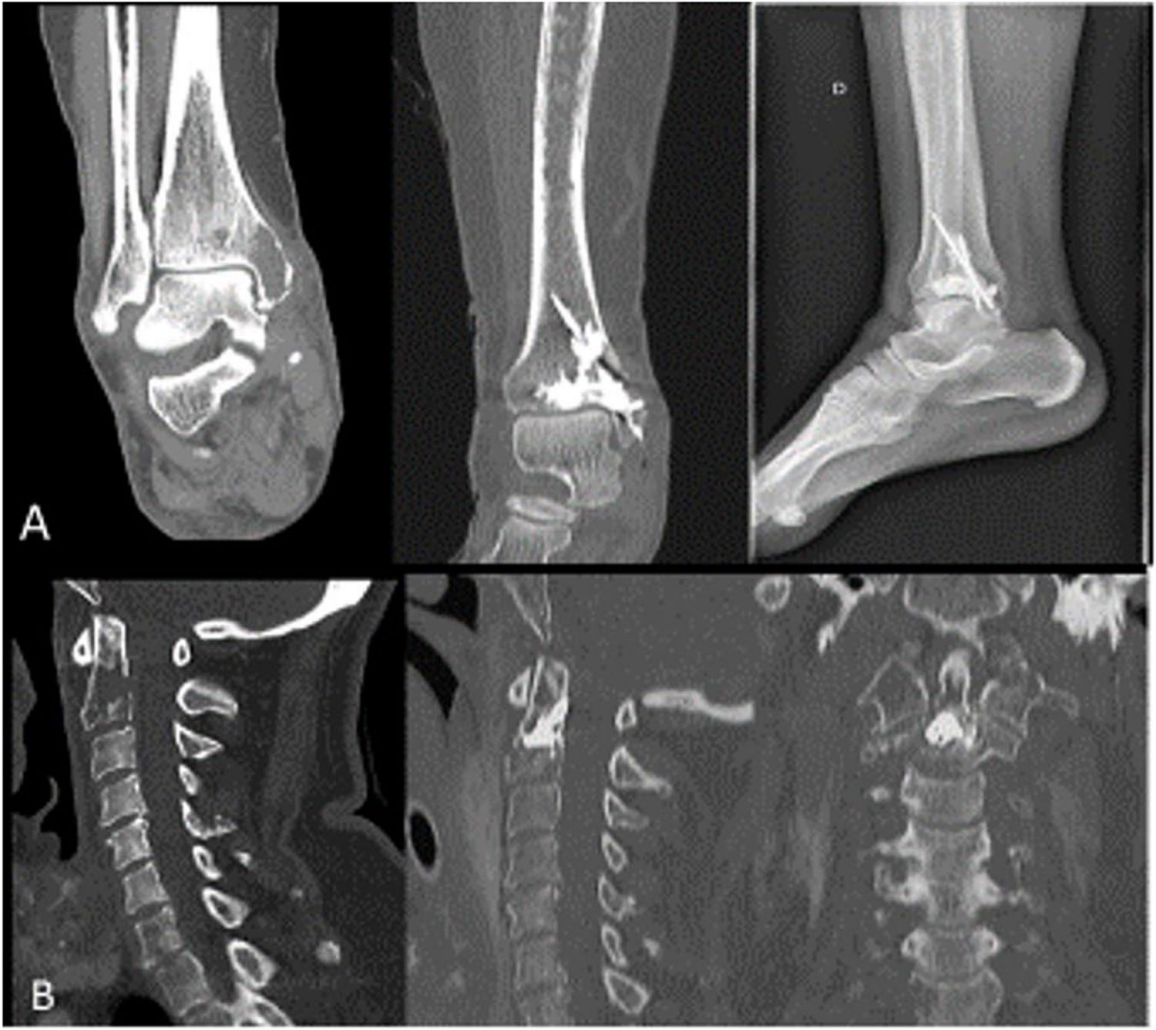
Examples of multimodal metastasis-directed therapy: A osteolytic lesion of the lateral malleolus of the right tibia with soft tissue involvement treated with radiotherapy, cementoplasty, and percutaneous osteosynthesis (left to right: coronal computed tomography (CT) scan of the lesion before any treatment; coronal CT scan of the right tibia after cementoplasty and osteosynthesis; sagittal radiography of the right ankle after treatment); B unstable osteolytic lesion of C2 treated with cementoplasty, screw fixation and radiotherapy (left to right: sagittal CT scan of the cervical spine with untreated lesion of C2; sagittal view after treatment; coronal view after treatment)
